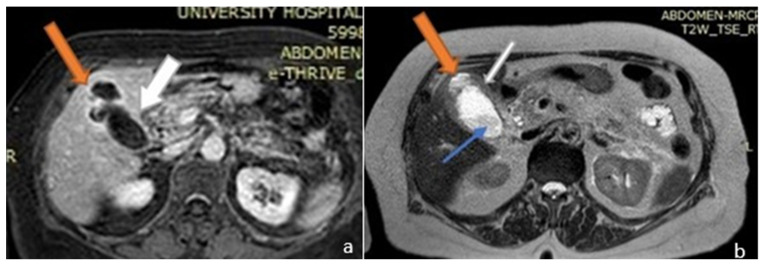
Magnetic resonance imaging (MRI) image of the upper abdomen. (a) Axial T1 post contrast and (b) T2. The gall bladder mucosa shows interrupted enhancement with a localized perforation anteriorly, close to the fundus. Orange arrows: liver abscess; white arrows: gallbladder; blue arrow: gallbladder stone.
